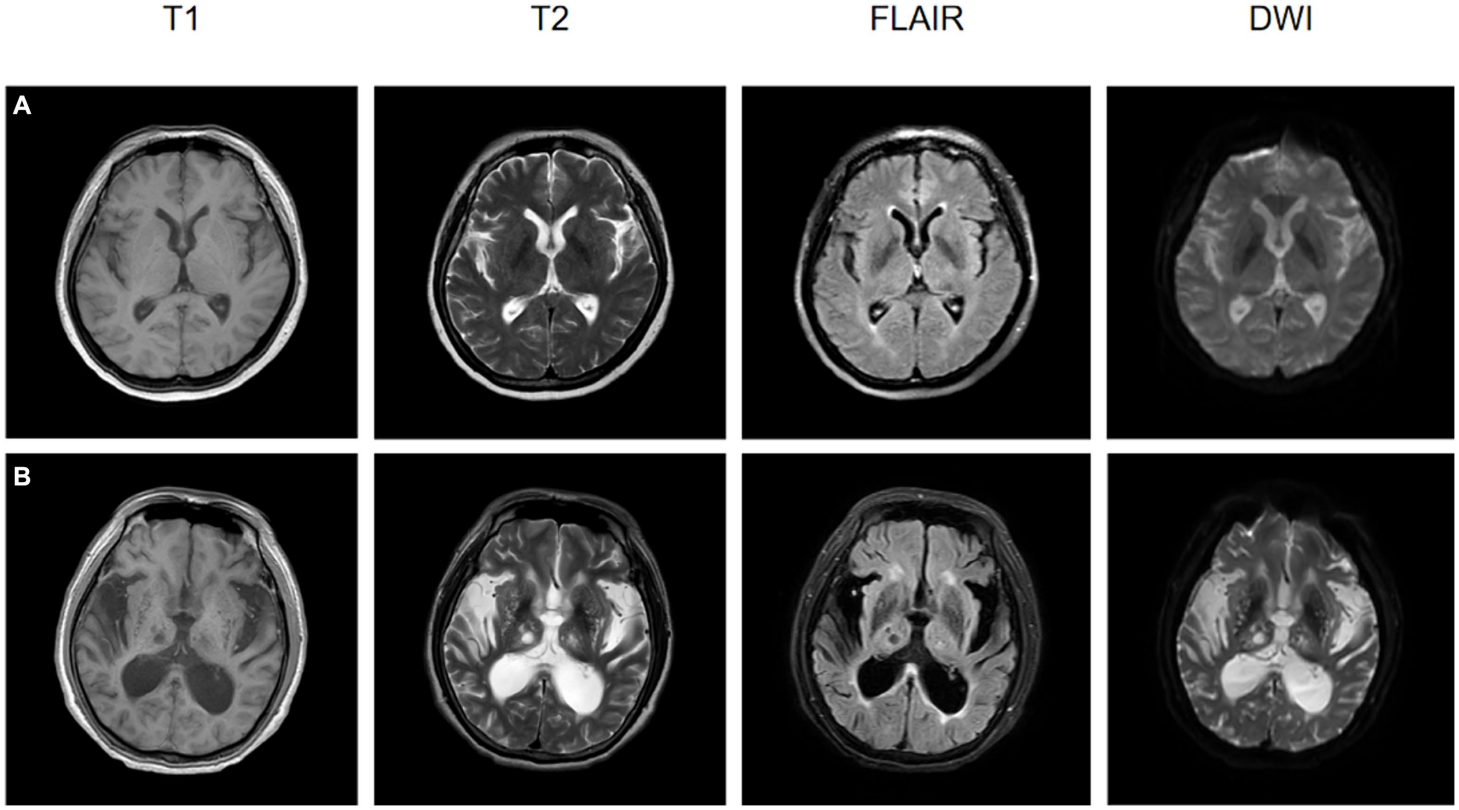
The MRI images at the onset of ischemic stroke for two patients, both of whom underwent rehabilitation training after their conditions stabilized, are presented. (A) A 57-year-old female patient, post-rehabilitation Berg Balance Scale score of 2, Functional Independence Measure (FIM) score of 6, and Modified Barthel Index of 3. (B) A 72-year-old male patient, post-rehabilitation Berg Balance Scale score of 0, FIM score of 1, and Modified Barthel Index of 0.
